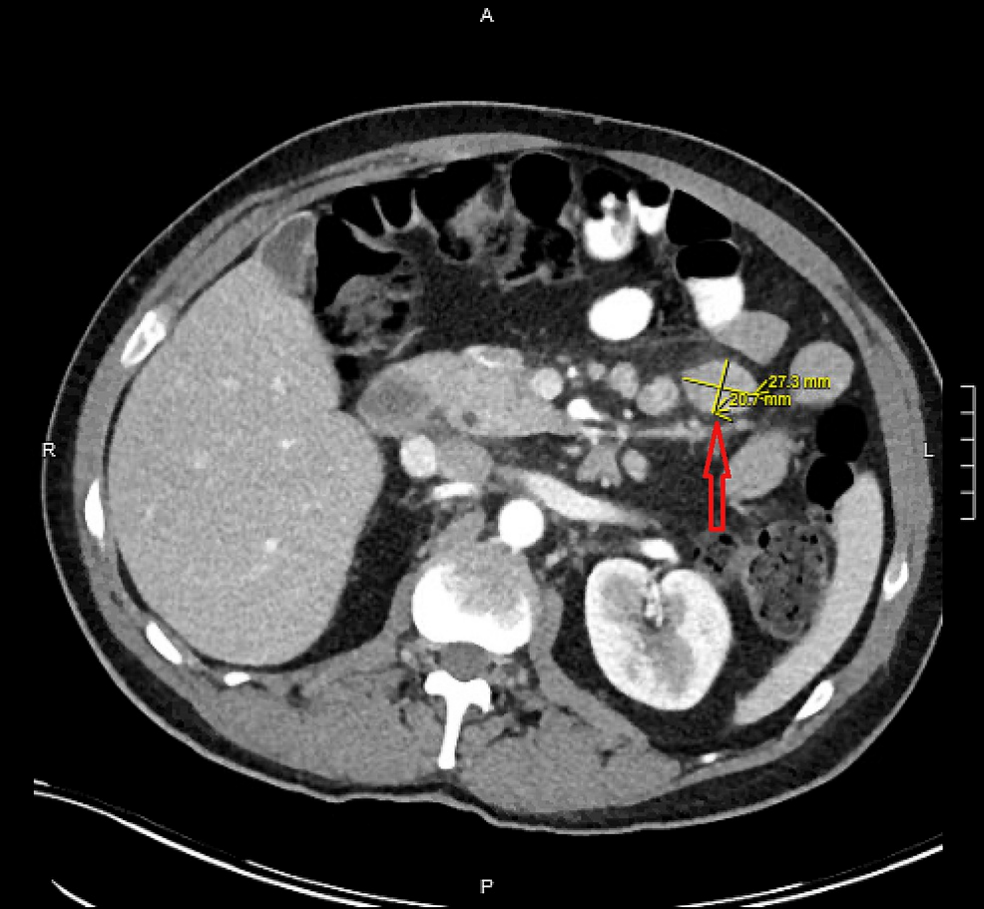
CT abdomen pelvis demonstrated enlarged mesenteric lymph node (red arrow), largest measuring 2.7x2.1 cm (yellow markings).
